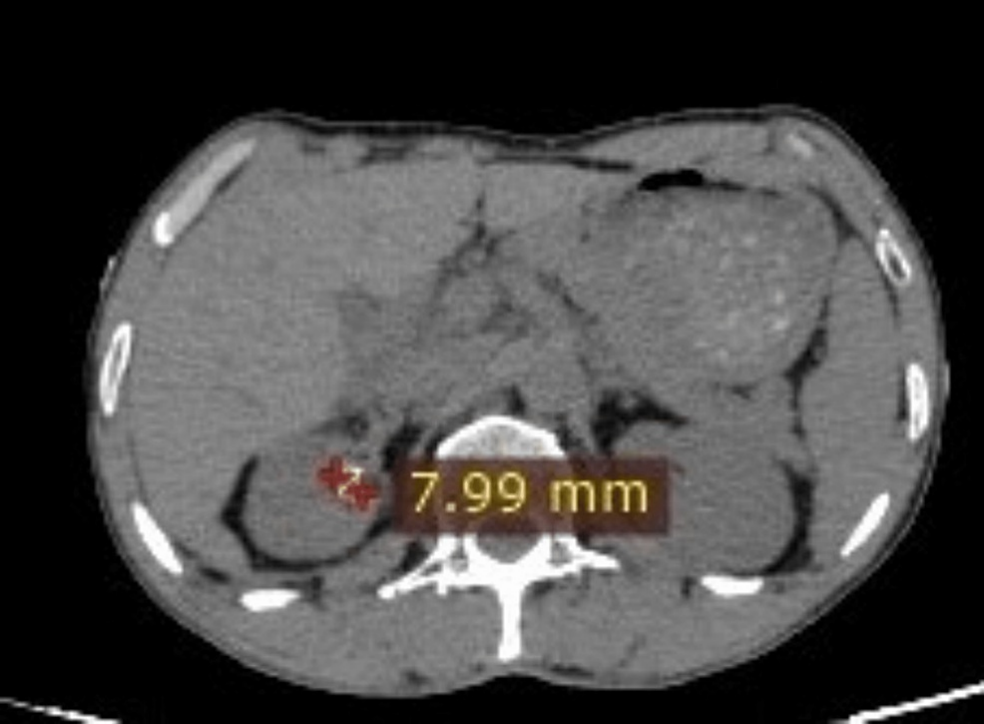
Axial CT section of the abdomen shows the measurement of a right renal calculus and its dimensions.
